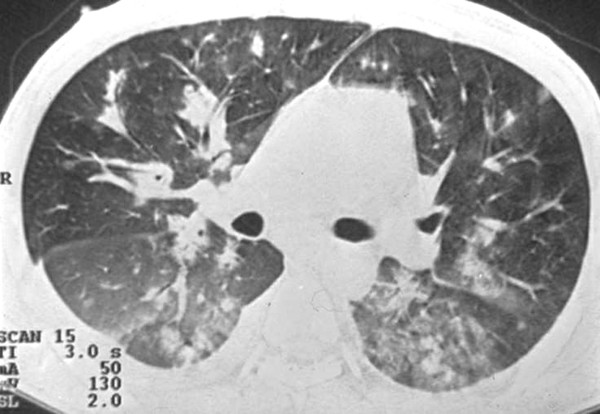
High-resolution CT scan of a patient with pulmonary KS at the level of the main bronchi shows ground-glass attenuation areas in the posterior regions of both lungs, which correspond to pulmonary hemorrhage. Peribronchovascular thickening is observed in the right lung, as well as bilateral pleural effusion.
